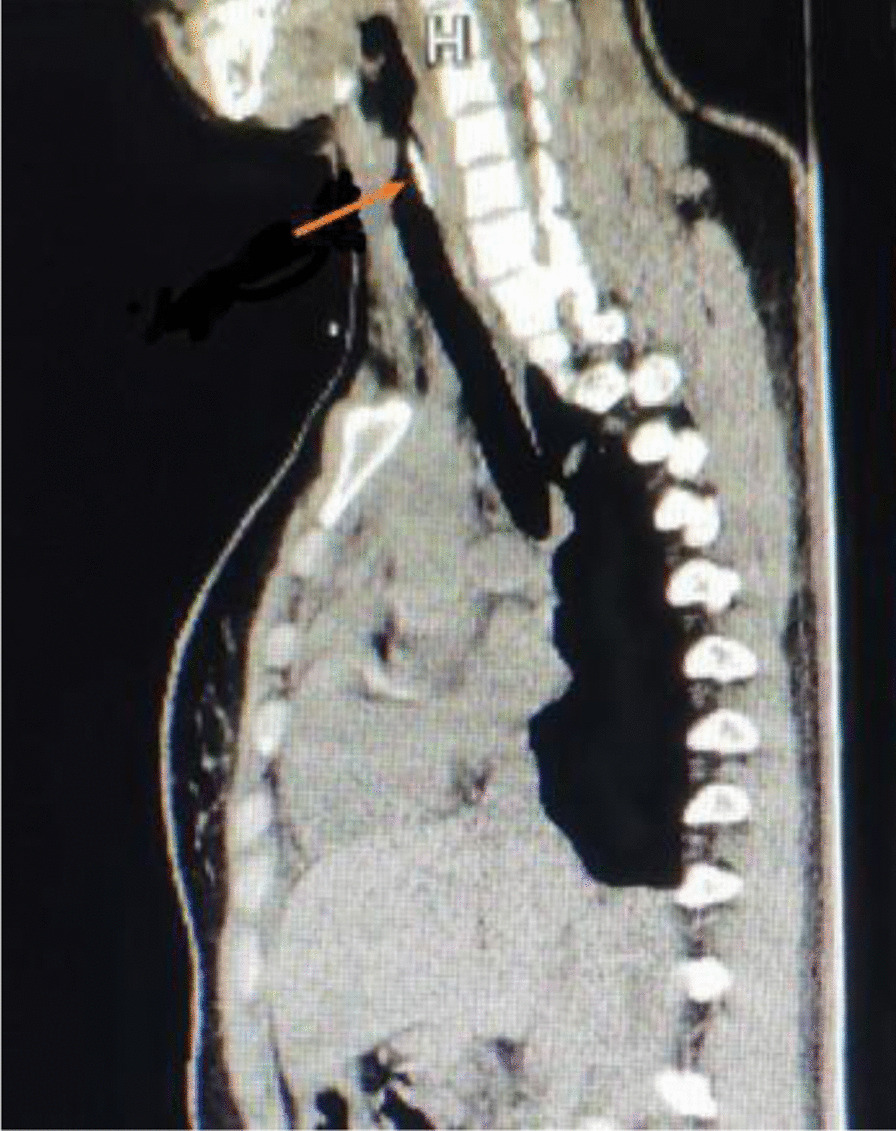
Sagittal reconstruction on the computed tomography images showed a metal spot (arrow) in the subglottic area
